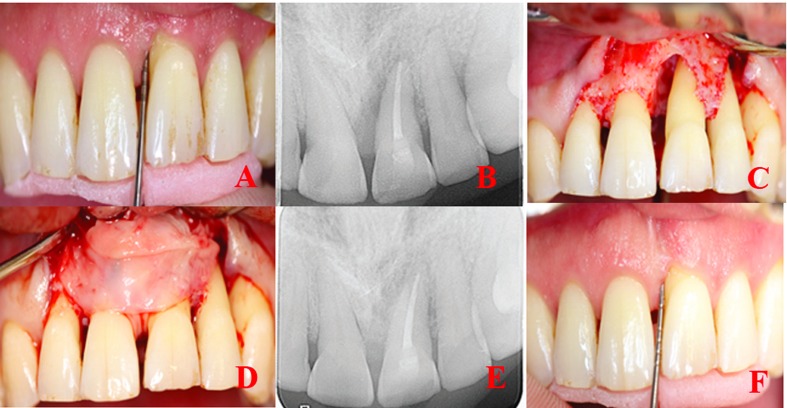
T-PRF procedure in the treatment of an intrabony defect with endo-perio lesion. A) Preoperative clinical view, B) Baseline radiograph, C) Intraoperative clinical image of typical intrabony defect, D) The defect is filled with the T-PRF membrane, E) Nine months postoperative radiograph, F) Nine months post-operative clinical view.
